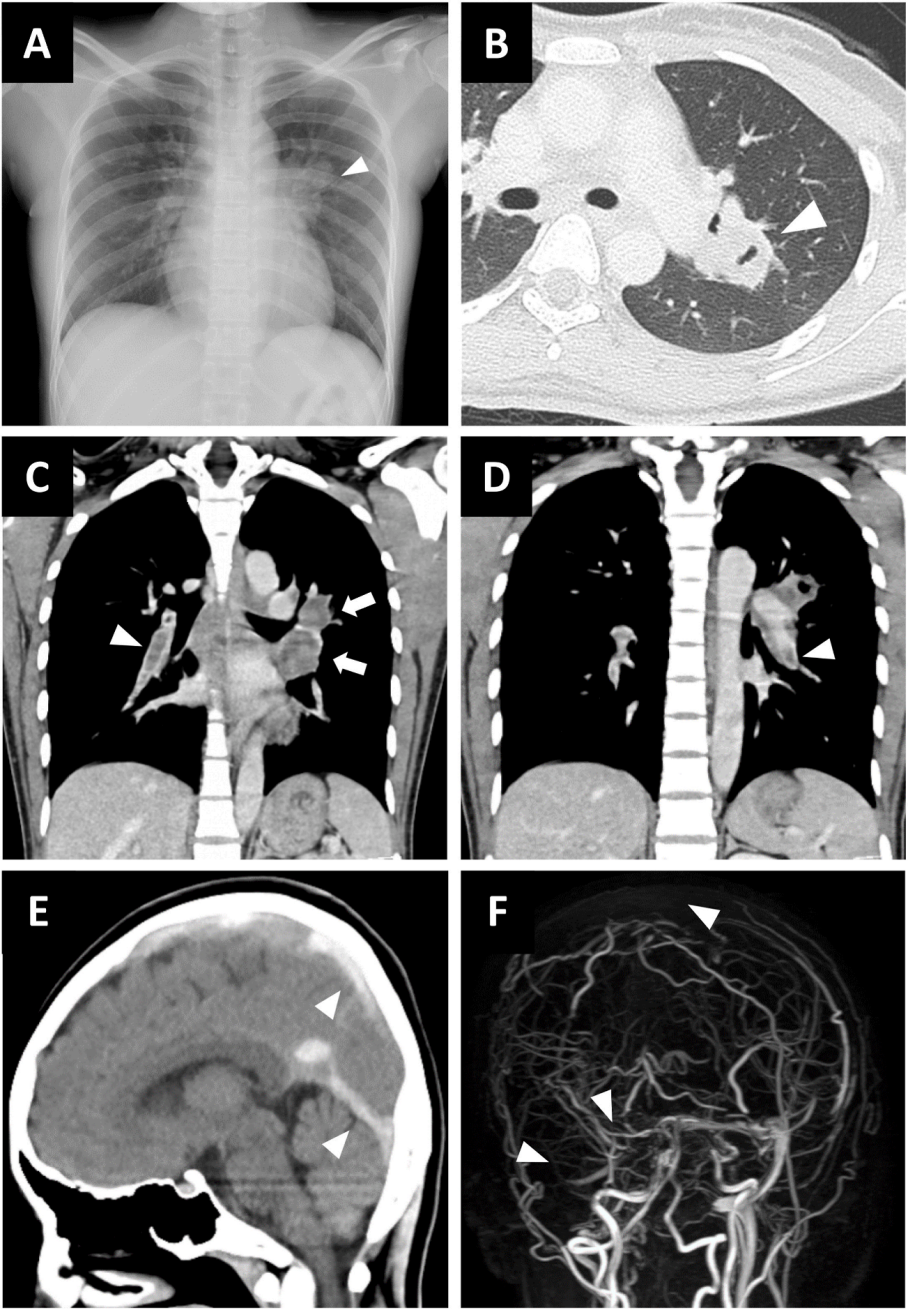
Radiological findings on admission A. Chest radiography showing a left hilar protruding shadow (arrowhead). B. Chest computed tomography (CT) indicating thick-walled lesions with cavities in the left upper lung (arrowhead). C-D. Contrast-enhanced CT of the chest revealing thrombosis in bilateral pulmonary arteries (arrowheads) and left hilar lymphadenopathy (arrows). E. Head CT (sagittal view) demonstrating high-density lesions in the superior sagittal and straight sinus (arrowheads). F. Magnetic resonance venography (MRV) of the brain. Loss of venous signals is seen in the superior sagittal, transverse, and sigmoid sinus (arrows), suggestive of acute thrombosis.
